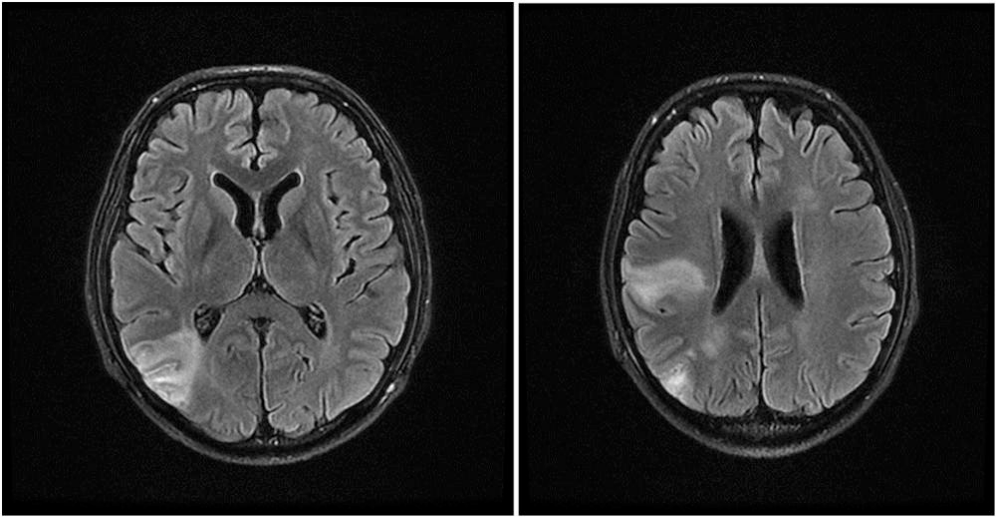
Patient's brain magnetic resonance imaging scan.
